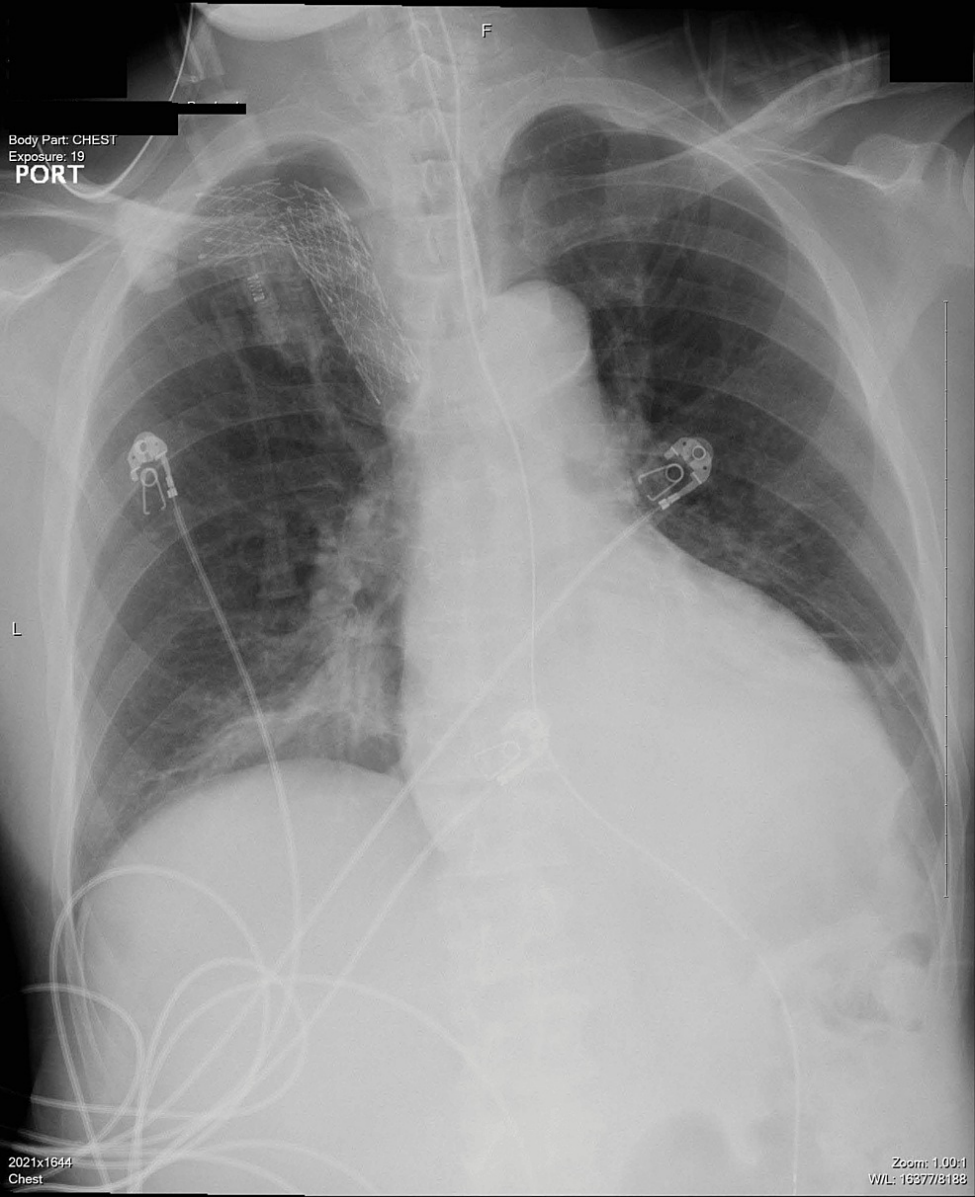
Chest X-ray revealed a new infiltrate in the posterior segment of the right upper lobe.
